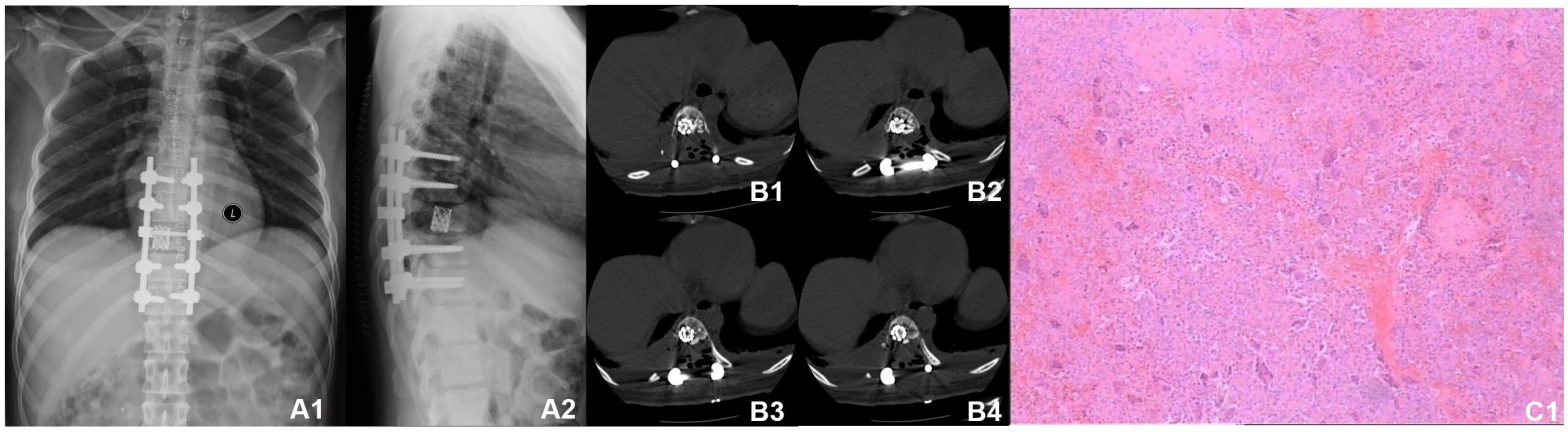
Postoperative radiographic data (2020-04). (A1,A2) Thoracic vertebral X-ray revealed a satisfactory internal fixation position; the placement was visible in the T9 vertebra. (B1–B4) Thoracic vertebral CT showing T9 vertebrae filled with a high-density shadow. (C1) Postoperative pathology revealed a T9 vertebral giant cell tumor of the bone.
